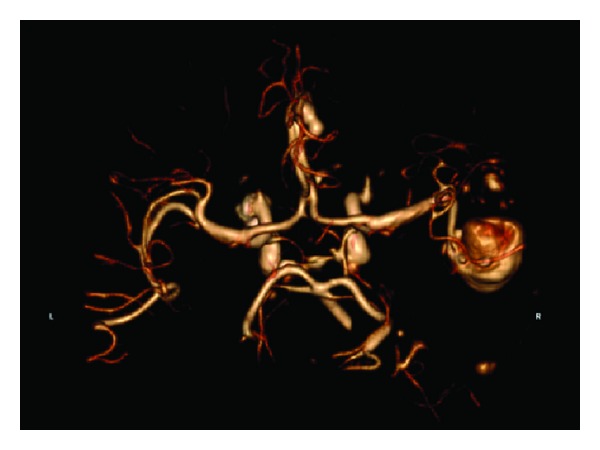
CT angio of the head showing two myxomatous fusiform aneurysms in the right middle (2.9 cm × 1.8 cm) and anterior cerebral arteries (10 mm × 5 mm).
